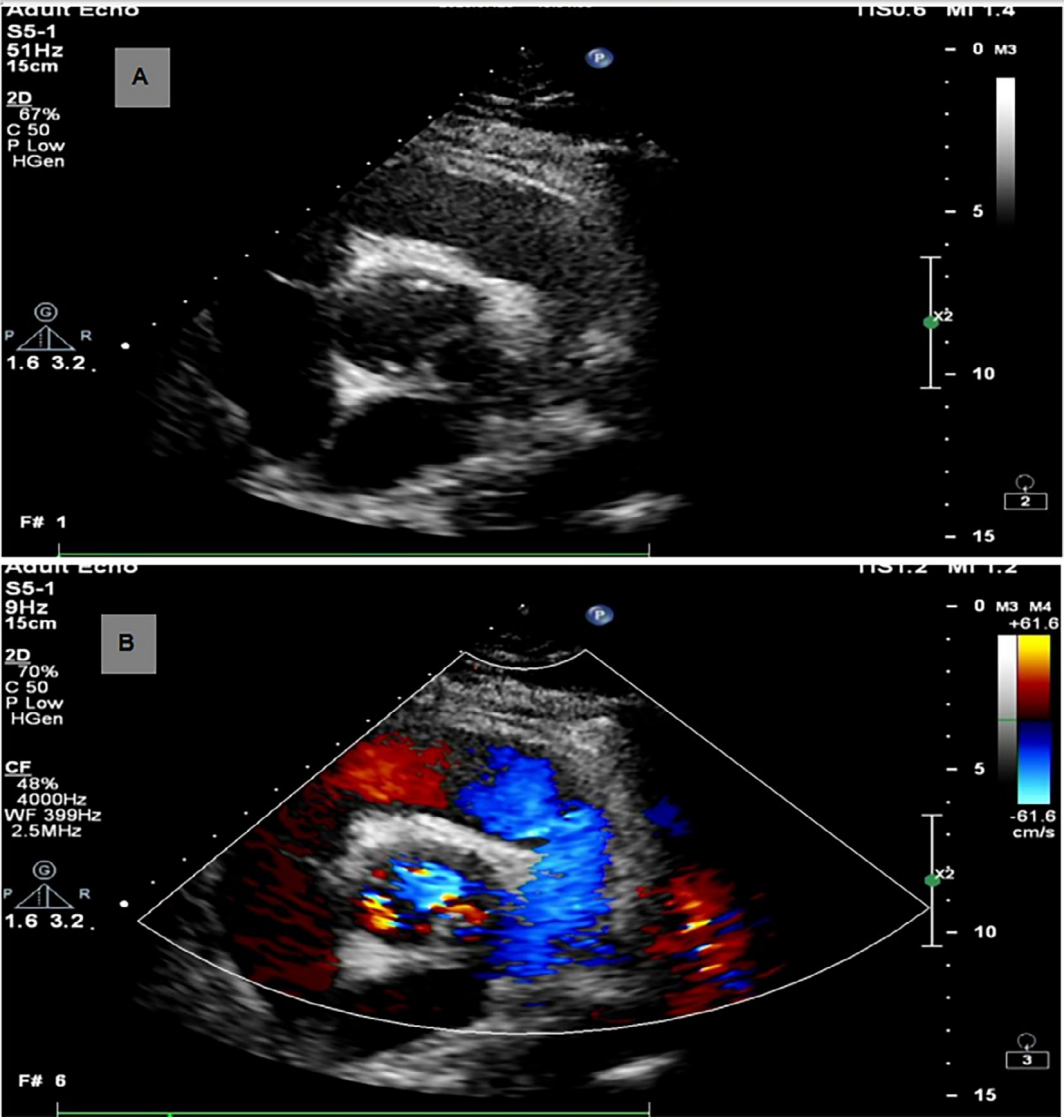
Transthoracic echocardiography imaging. (A) Aortic valve short axis. (B) Aortic valve short axis with color‐flow Doppler.
